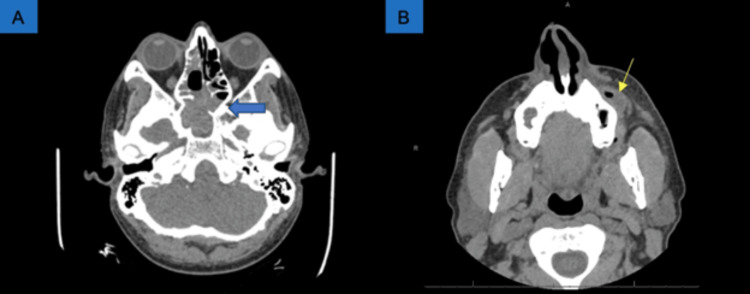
Repeat CT maxillofacial on day 7 of admission (A) Postsurgical changes with partial ethmoidectomy and sphenoidectomy. Soft tissue obstruction of contralateral left sphenoid sinus (blue arrow); (B) Abscess of #12 (yellow arrow is enhanced over radiologist’s indicator).
